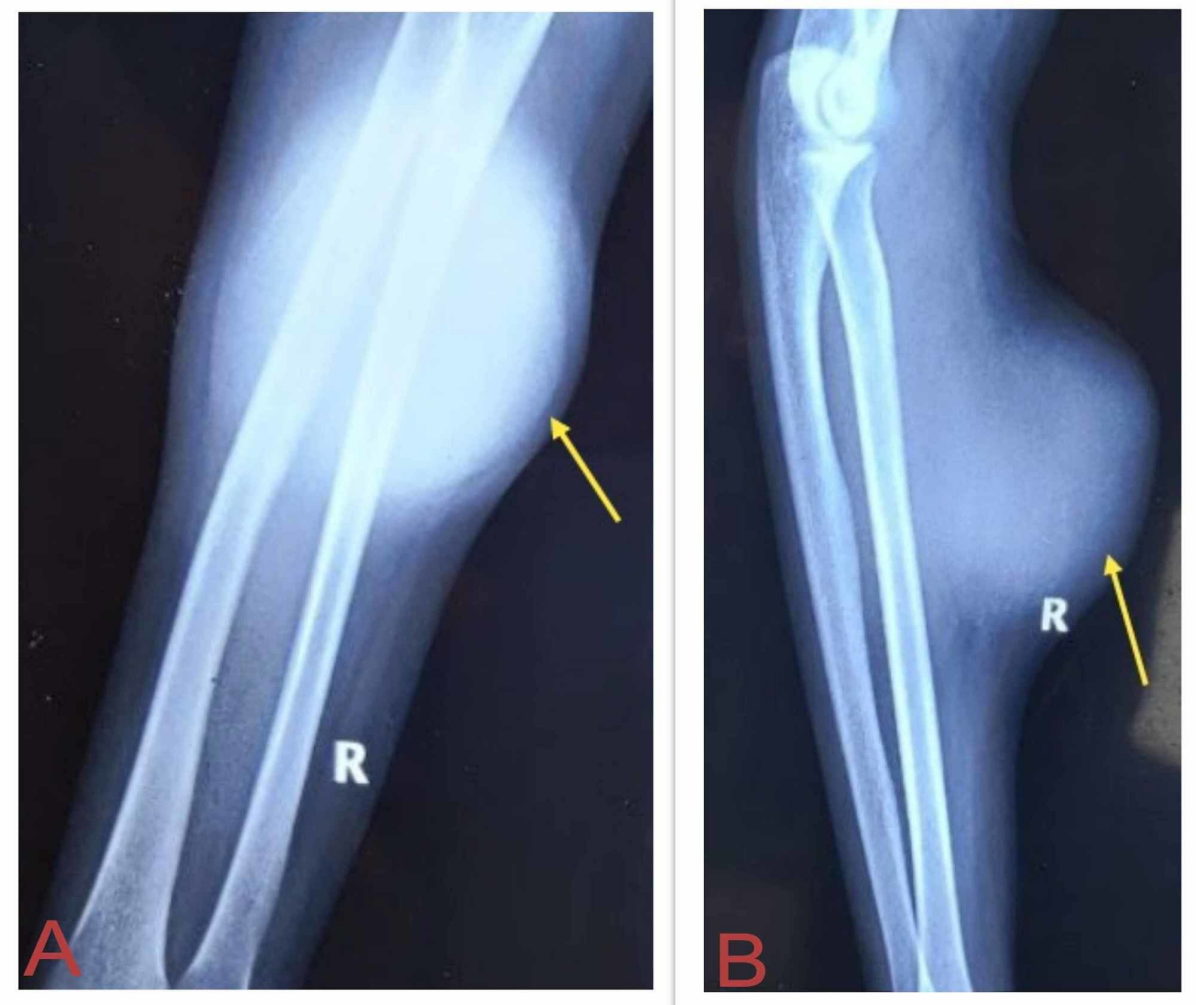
Preoperative X-rays of the right forearm showed increased soft tissue shadows with no bony involvement. (A) Anteroposterior view. (B) Lateral view.
